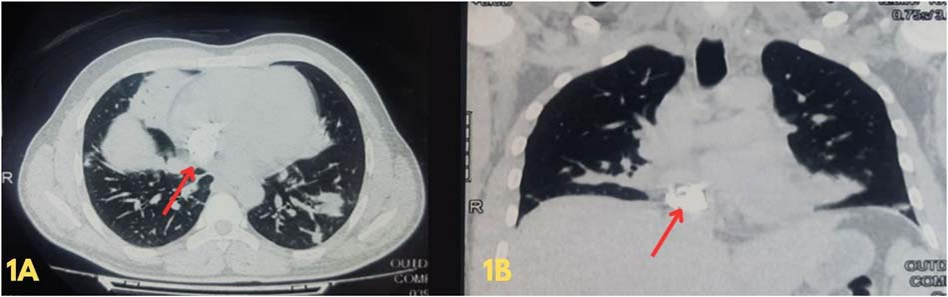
(A and B) Axial and coronal views of a high-resolution computed tomography scan of the chest showing multiple opacifications in the lung field and heavy calcification within the right atrium, extending up to the opening of the inferior vena cava.
